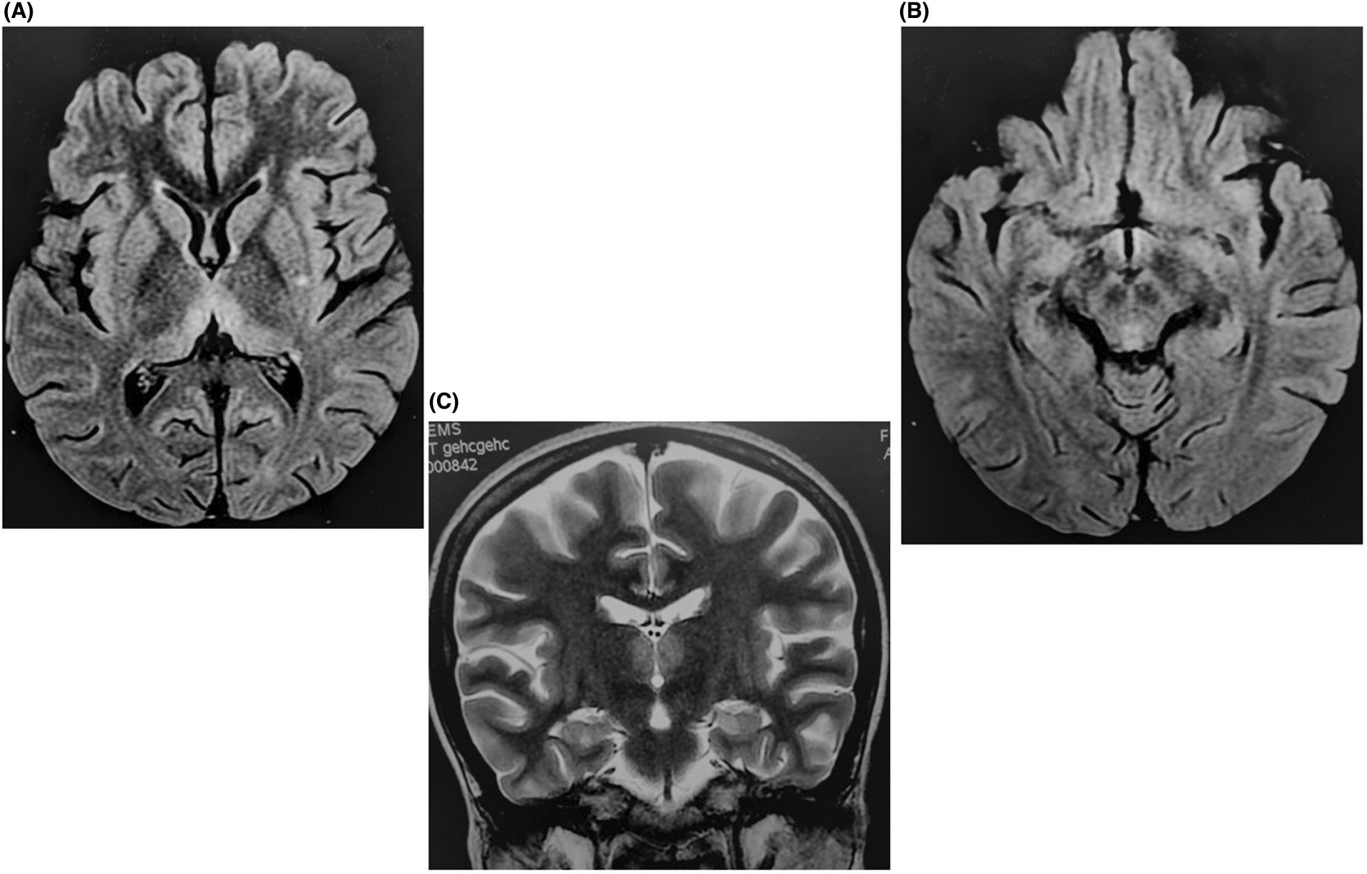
(A) showing hyperintensities on FLAIR images in bilateral medial thalami. (B) showing hyperintensities on FLAIR images in periaqueductal gray matter. (C) showing hyperintensities in bilateral mamillary bodies.
